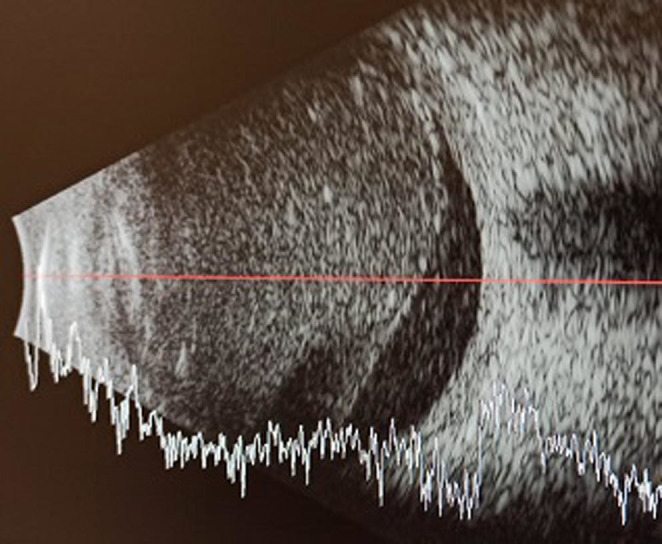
B-scan ultrasonography showing dense opacity throughout the vitreous cavity with attached retina
